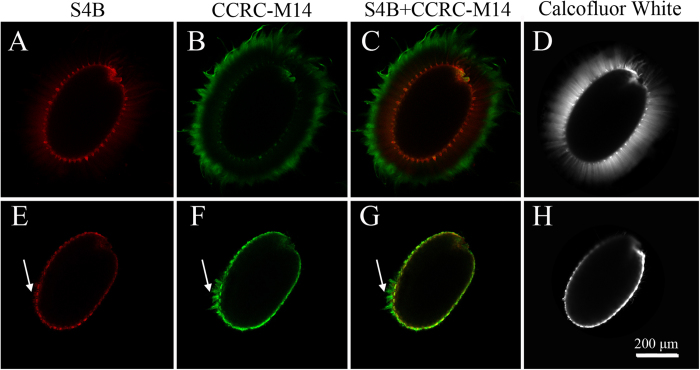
Visualization of mucilage before (A–D) and after 20 seconds of ultrasonic treatment (E–H). The arrow shows the cellulose and RG-I residues after 20 seconds of ultrasonic treatment. Scale bar: 200 μm.
